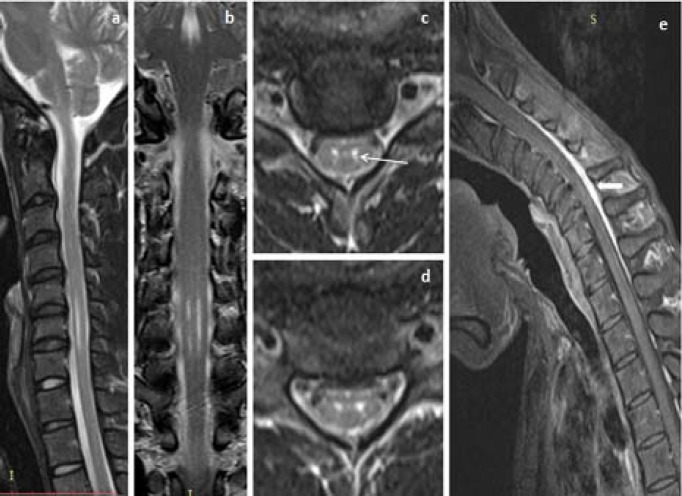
The same patient in figure 1, neutral position sagittal and coronal T2WI MRI images (a, b) showed segmental hyperintensities in lower cervical cord extending from C5 to C7 vertebral level, axial T2WI (c, d) showed symmetrical hyperintense signal in AHC (white arrow), post-gadolinium fat suppressed flexion MRI image (e) showed enhancing crescent shaped posterior epidural lesion extending from C4 to T2 vertebral level (block arrow)
